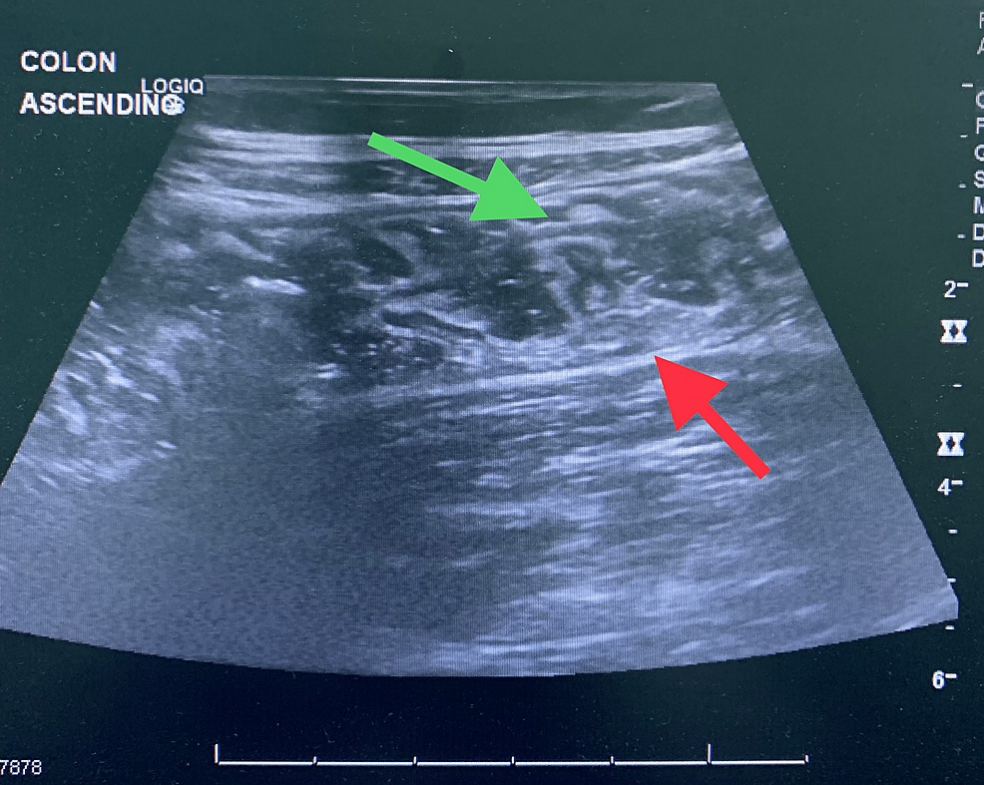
Ultrasound image of Behcet's colitis: thick-walled (red arrow) ascending colon with edematous mucosa (green arrow).
